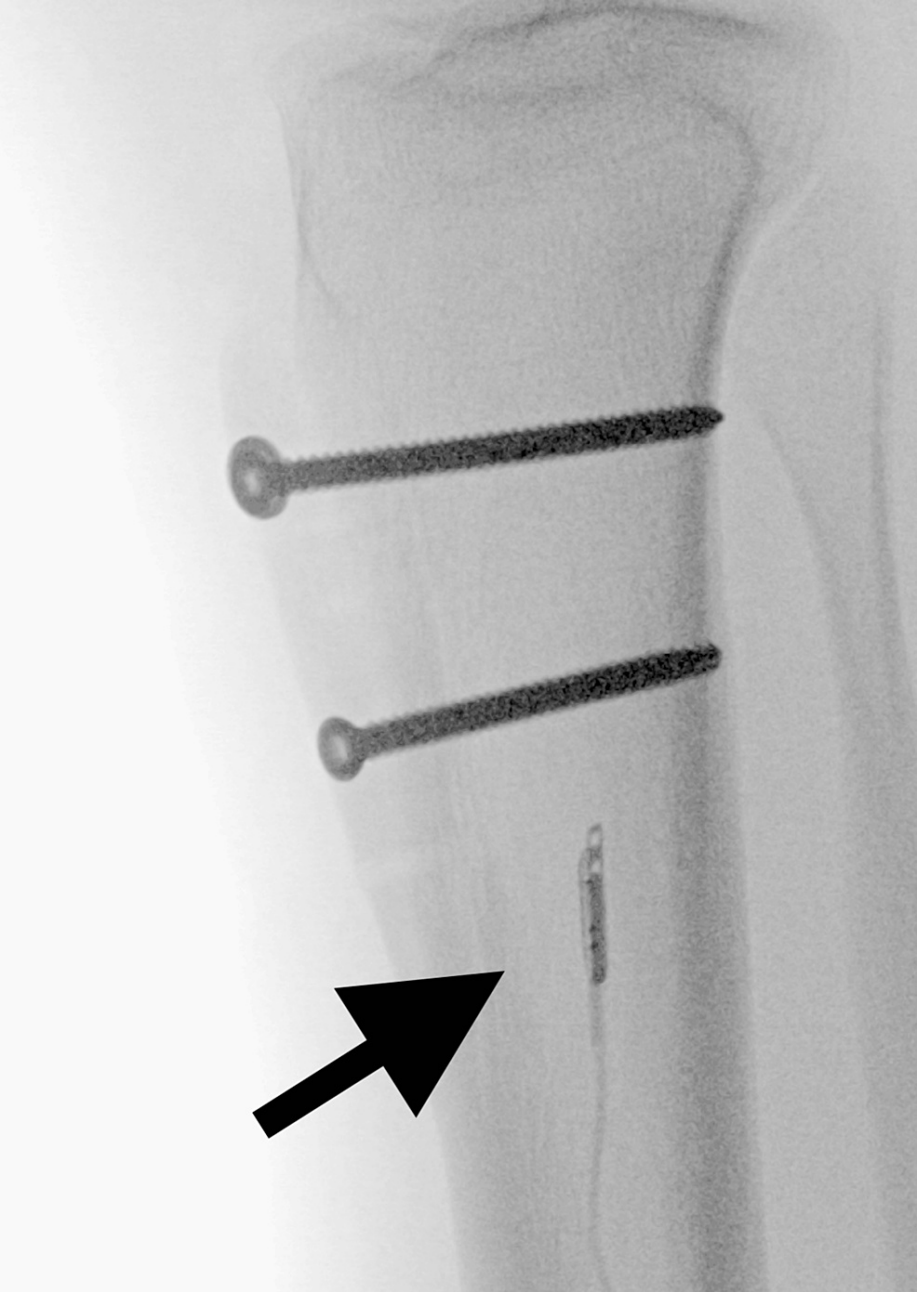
Lateral radiograph of the previous operative site. Lateral radiograph taken in the operating room shows no displacement of the fixation or breakage of hardware. Arrow shows sensor in good place in the muscle belly.
